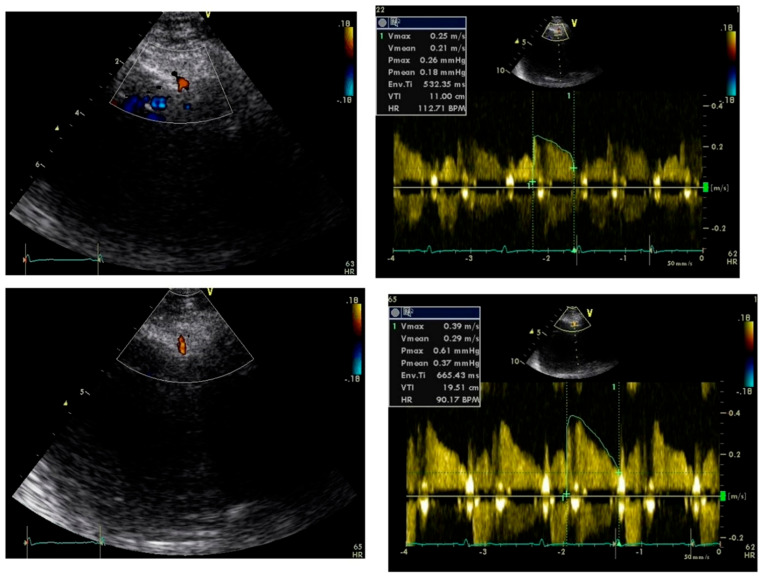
Coronary microvascular assessment using transthoracic Doppler. Transthoracic Doppler echocardiography of the left anterior descending artery (LAD) at rest (upper-left) and during hyperemia (lower-left), demonstrating diastolic-dominant coronary flow velocity patterns. The combined Doppler recordings yield a CFR < 2, consistent with significant stenosis of the left anterior descending artery.
